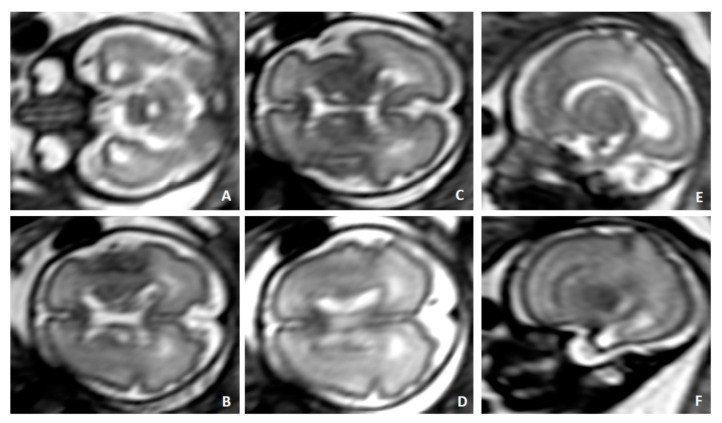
Fetal MRI, 30 GW of Case 2; SSHTSE (Single Shot Turbo Spin Echo) sequences and transverse (A–D) and sagittal images (E,F). Typical temporal pole abnormalities: temporal horn enlargement with cystic appearance (A–F), ventricular septation and white matter hyperintensity. Diffuse white matter hyperintensity. Multiple subependymal cysts.
